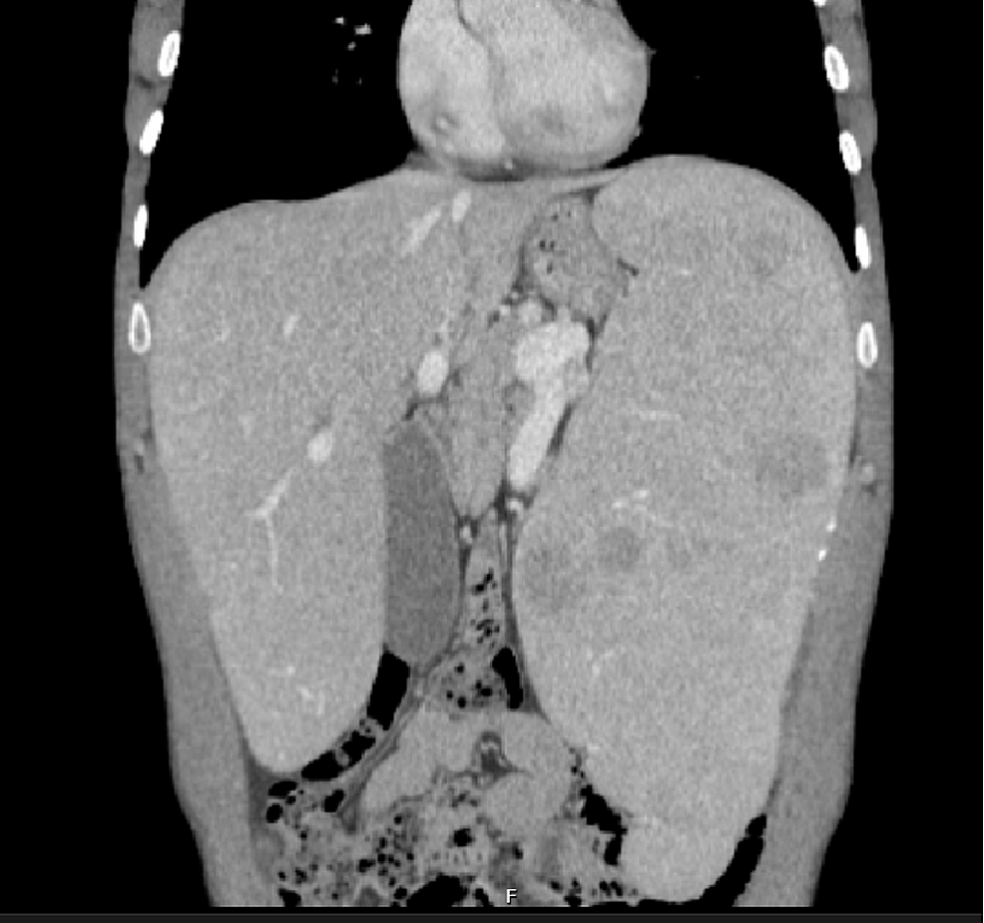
CT scan showing hepatosplenomegaly.
